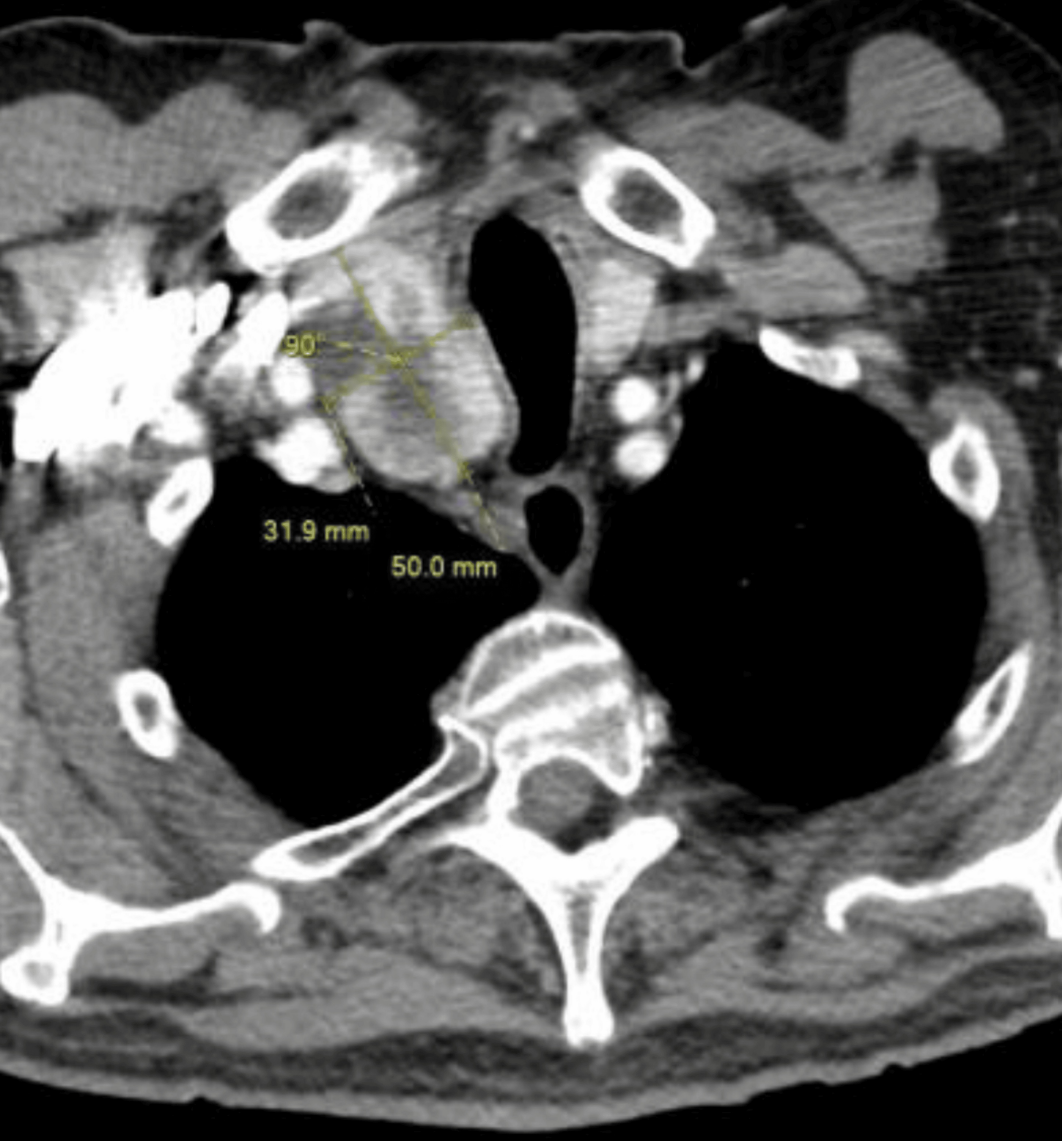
Thyroid goiter on CT chest Official read of thyroid: The right thyroid is severely enlarged and heterogeneous, mildly displacing the trachea leftward.
